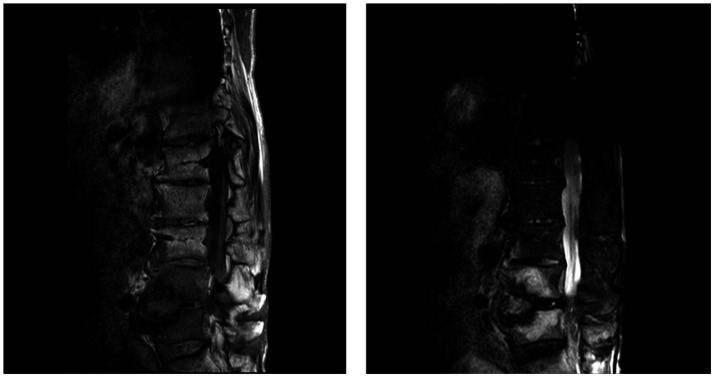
MR images of lumbosacral vertebrae.
